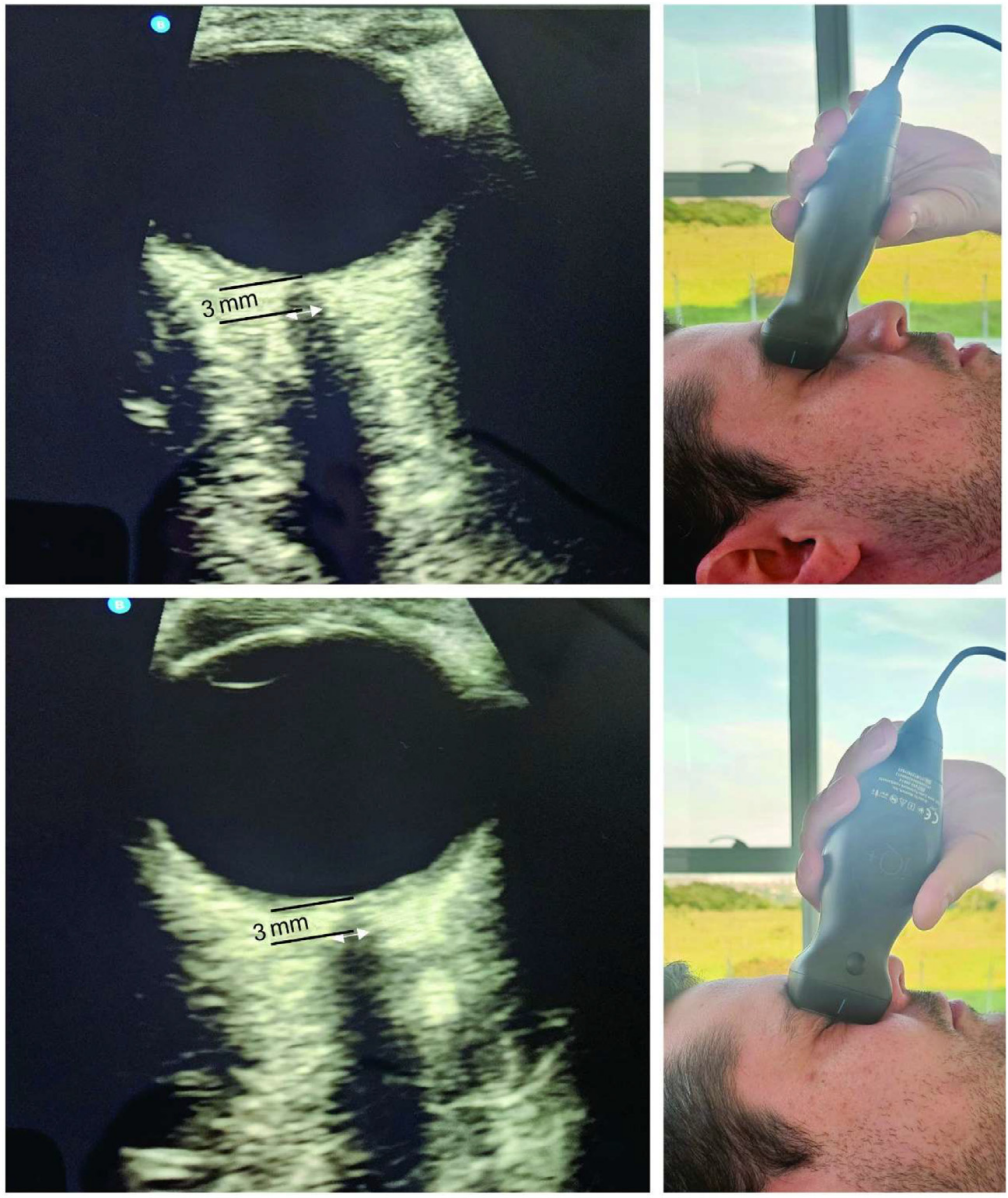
Ocular ultrasound: 3 views [transverse (top), sagittal (middle) and bottom (bottom)], diameter of red optic nerve sheath (inside diameter), diameter of yellow optic nerve sheath (outside diameter), green distance 3 mm behind the globe (Kishk and Ebraheim, 2019; Raval et al., 2020).
